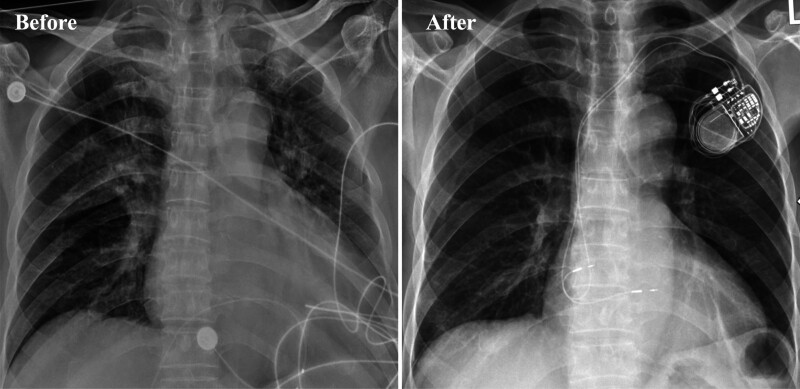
Chest X-ray before operation and at 1-yr follow-up.
